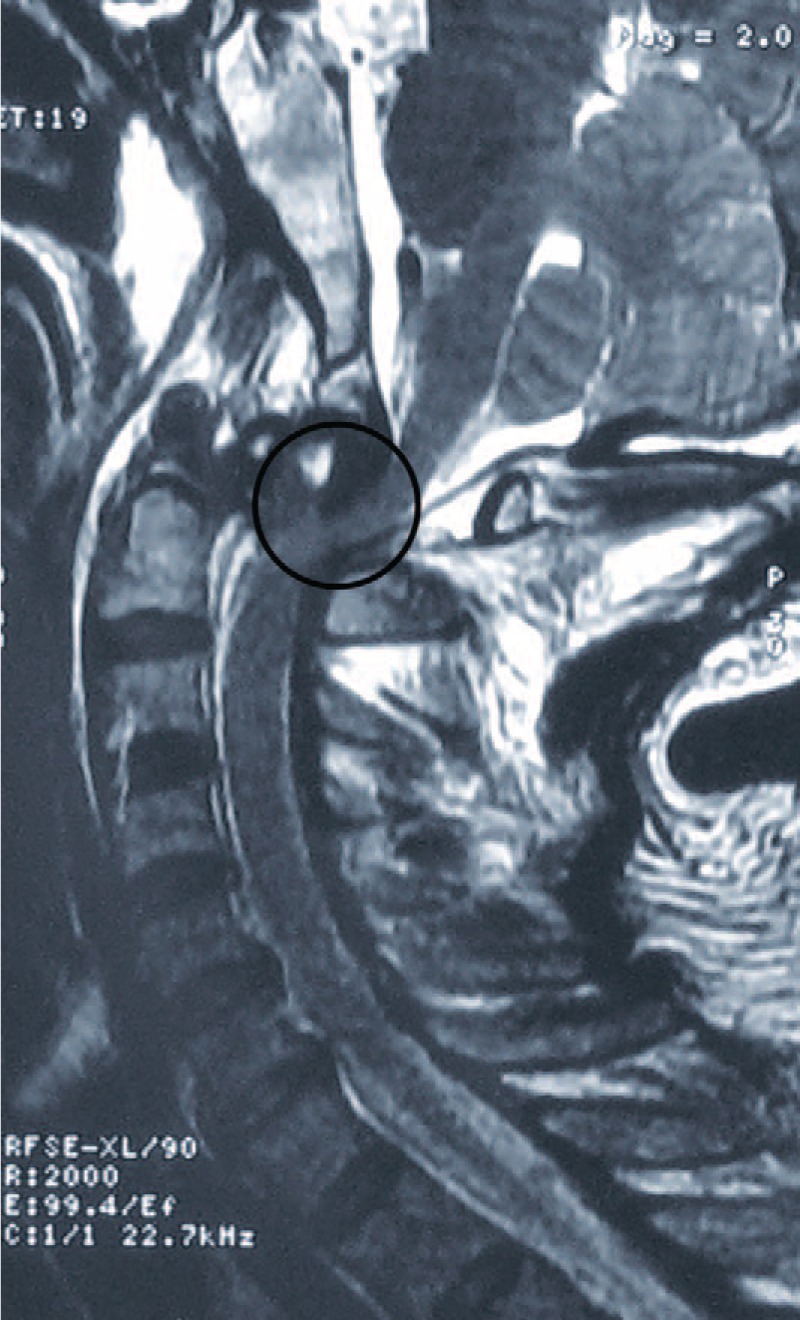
MRI showed obvious spinal cord displacement with devious course. The obliteration of the subarachnoid space and inconspicuously mild cord compression (the black circle) are demonstrated at the level of atlantoaxial dislocation. MRI = magnetic resonance imaging.
